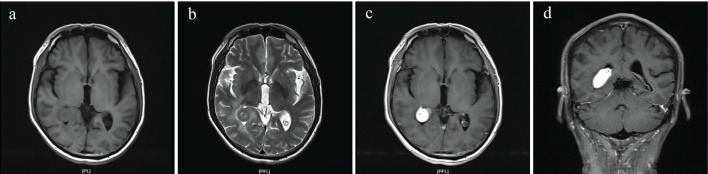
Magnetic resonance imaging (MRI) images showing a mass-like lesion in the right lateral ventricle with isointense signal on T1-weighted imaging (T1WI) (a), mixed high and low signal on T2-weighted imaging (T2WI) (b), and marked contrast enhancement on coronal (c) and axial planes (d).
